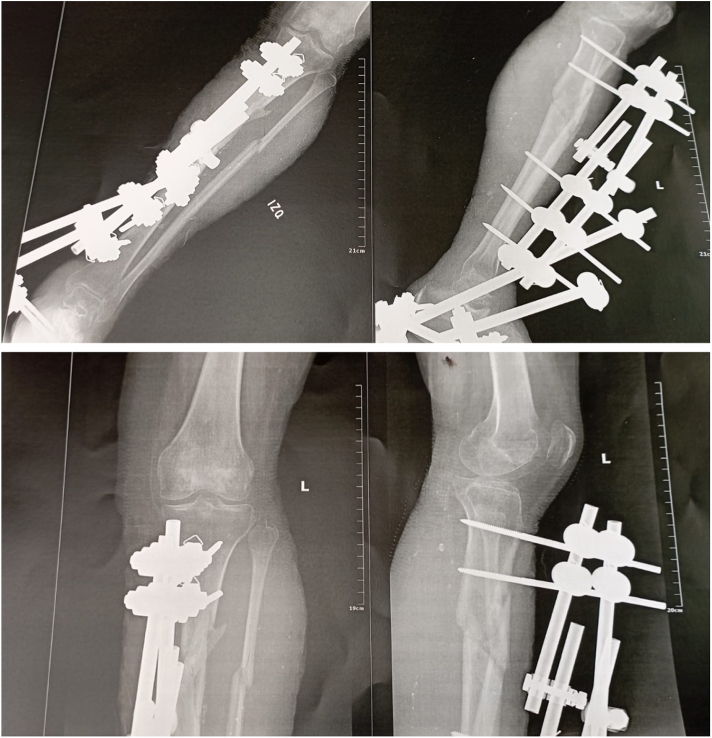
AP and lateral x-ray of the left leg and knee observing callus formation of the tibia and fibula.
